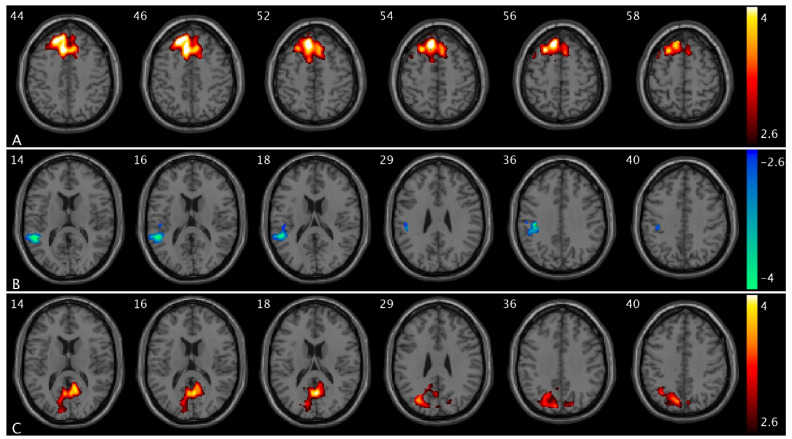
Statistical maps overlaid on T1-weighted images showing altered CBF from low- to high-urge state in healthy controls and OAB participants. (A) CBF increases in the superior frontal, superior medial frontal, anterior cingulate, and supplementary motor regions in healthy controls; (B) CBF decreases in the supramarginal, middle, and superior temporal regions in healthy controls; (C) CBF increases in the precuneus, cuneus, and superior occipital regions in OAB participants from low- to high-urge state. The numbers in the top left corner of the MRI images indicate the z-coordinate (in mm) in the MNI space. The color bars represent t-values.
